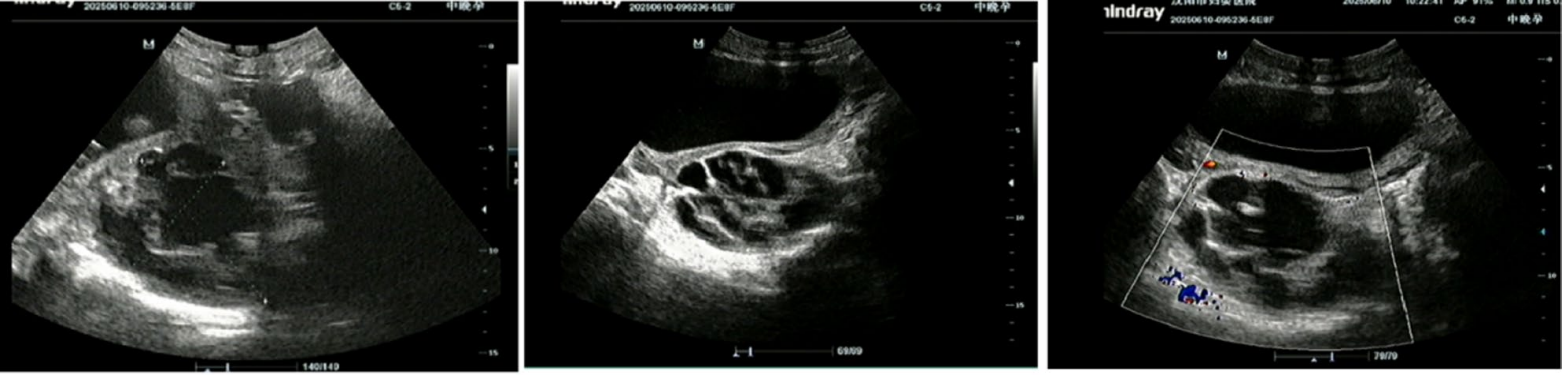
Transvaginal ultrasound on admission at 33 + 5 weeks of gestation. A cystic mass (87 mm × 68 mm × 67 mm) with internal septations is seen posterior to the cervix
